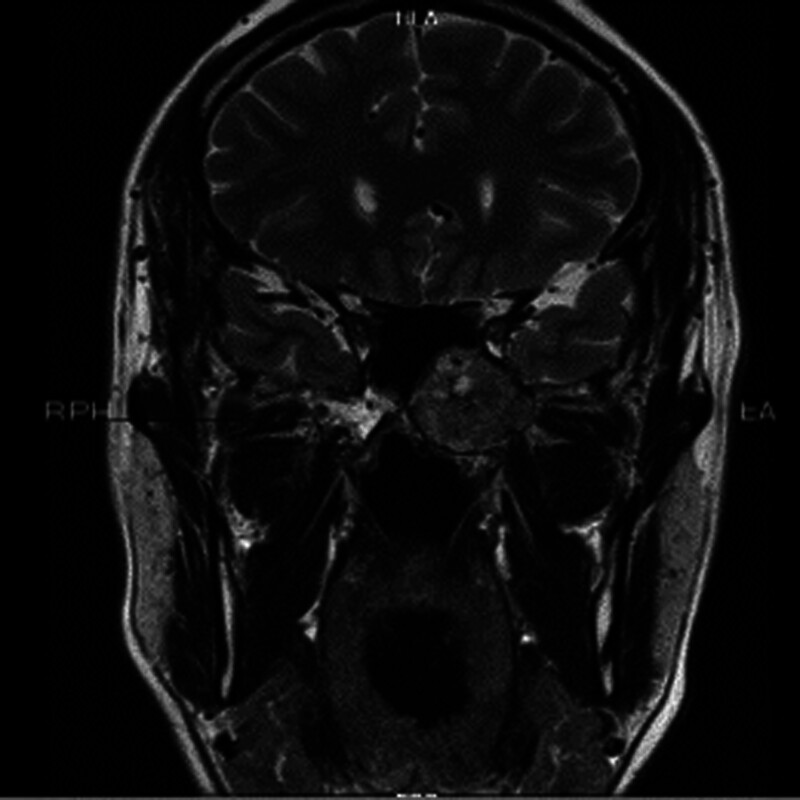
A 54-year old male patient with skull base schwannoma. Sinus MRI coronal view. MRI = magnetic resonance imaging.
